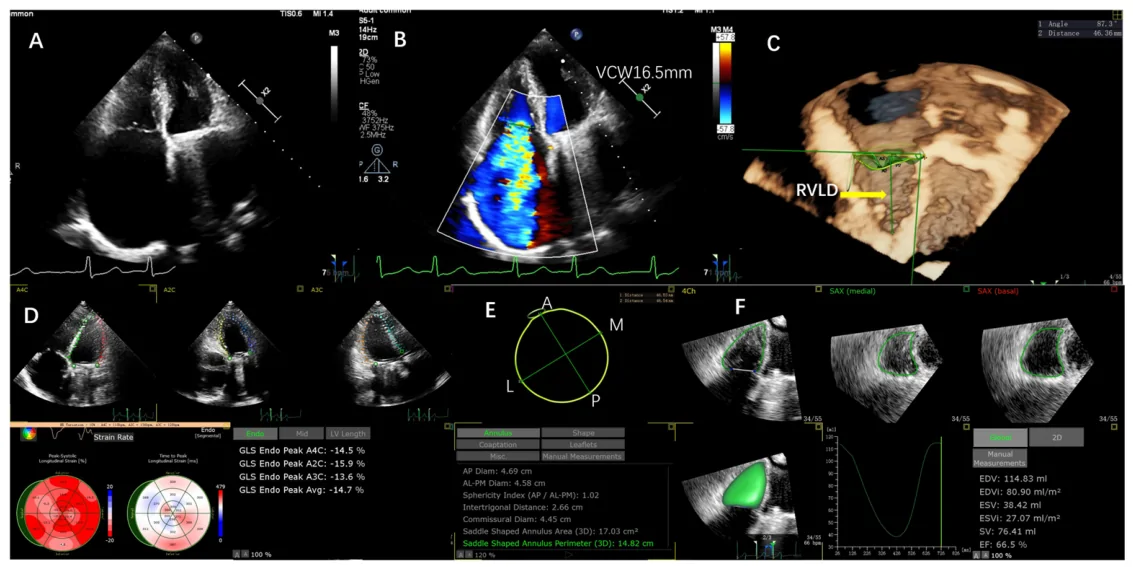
Two-dimensional and three-dimensional echocardiographic measurements. (A). Apical four-chamber view of a patient 21 years after mitral valve replacement; (B). Tricuspid regurgitation graded as 5, with a vena contracta width (VCW) of 16.5 mm; (C). Right ventricular longitudinal diameter (RVLD) measured using three-dimensional echocardiography; (D). Strain analysis used for ventricular functional assessment; (E). Tricuspid valve anteroposterior diameter (TVAPD) measured using a three-dimensional software package; (F). Right ventricular end-systolic volume index (RVESVI) measured using a 3D automated RV analysis software package.
